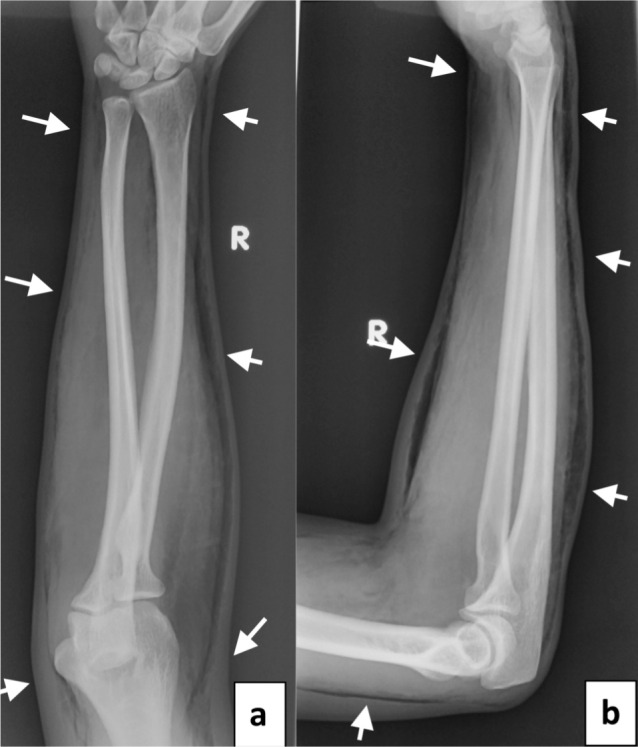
a and b: Antero-posterior and lateral radiograph of the right forearm showing subcutaneous emphysema limited to the subcutaneous plane, extending to the dorsum of hand and posterior aspect of arm.
